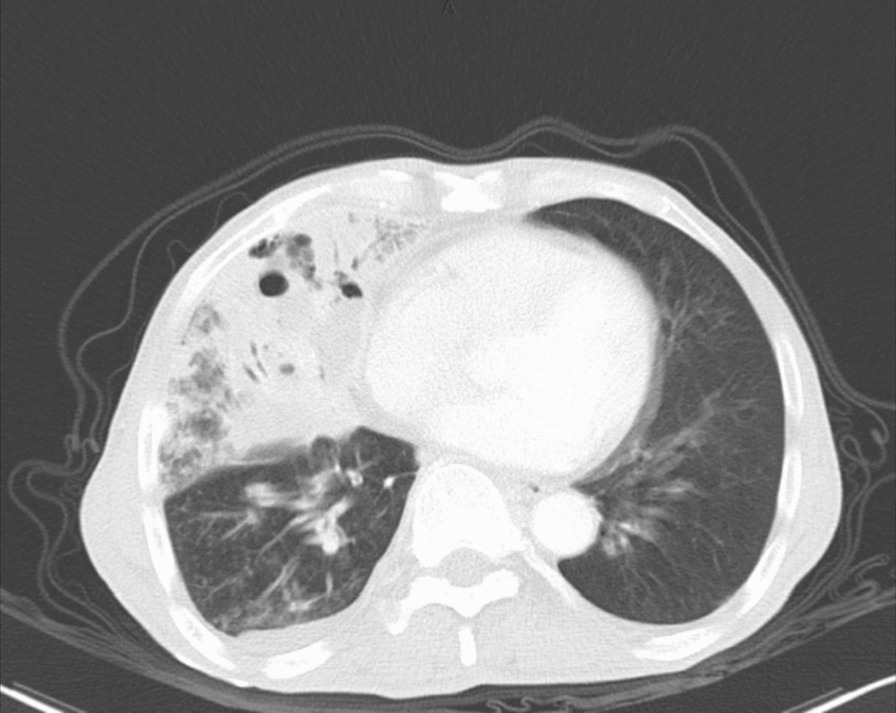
Chest CT scan showing sizable solid shadow in the middle and lower lobes of the right lung, large right pleural effusion with incomplete expansion of the right lower lung under pressure
